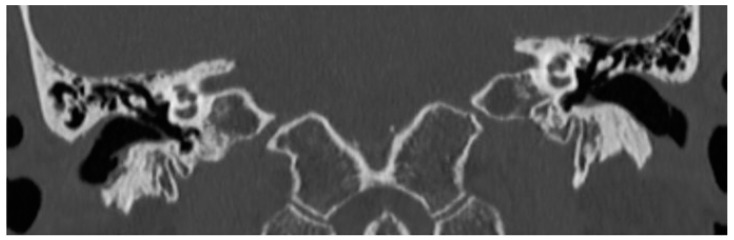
Patient 2, CT scan: evidence of a thin layer of soft and erosive tissue on the right inferior wall of the EAC.
